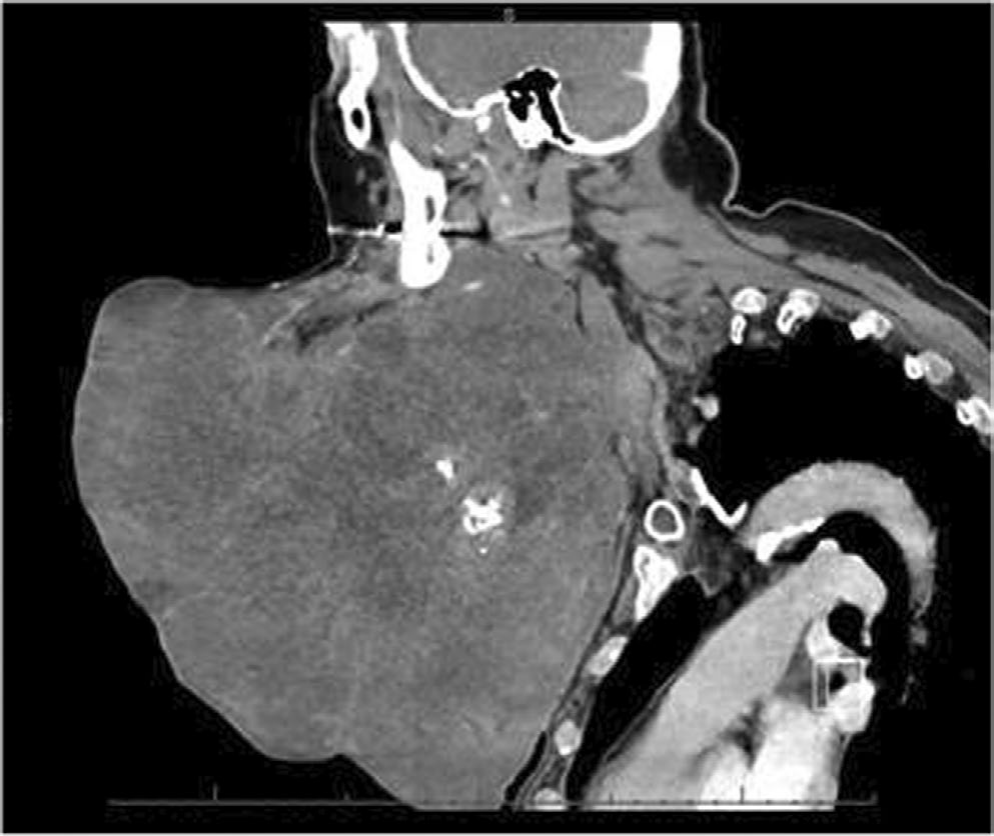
Sagittal CT image of the neck mass at presentation.
